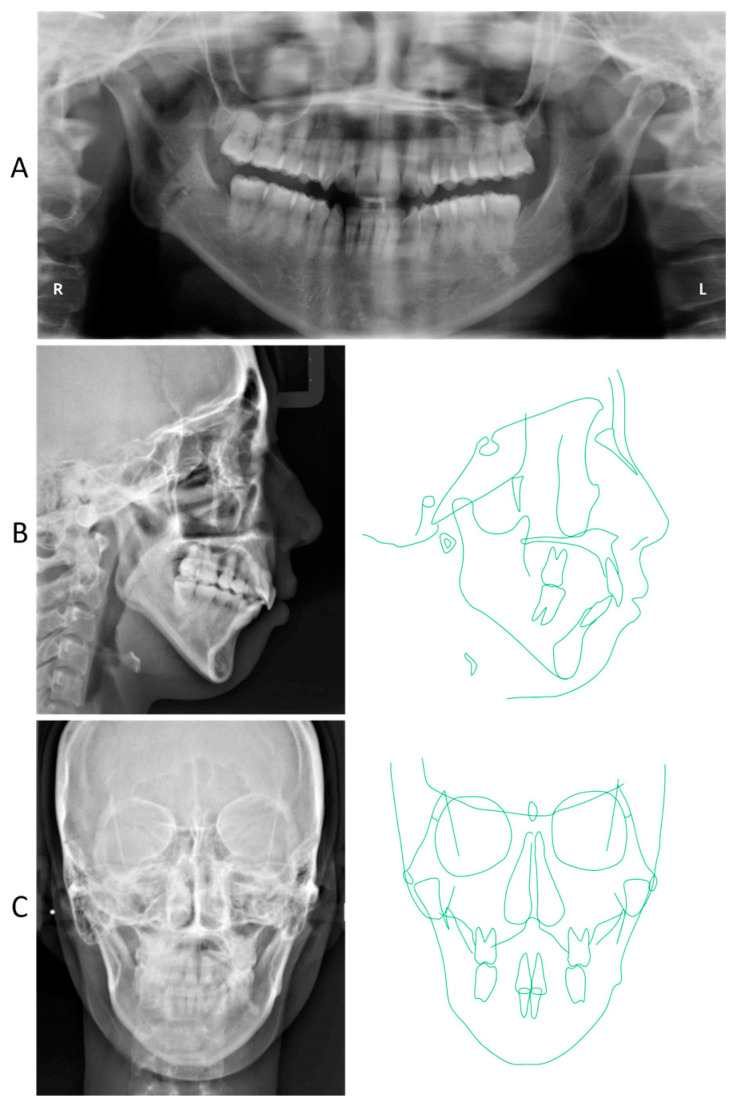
Postretention radiographical records. (A) Panoramic radiograph; (B) Lateral cephalogram and its tracing; (C) Frontal cephalogram and its tracing.
